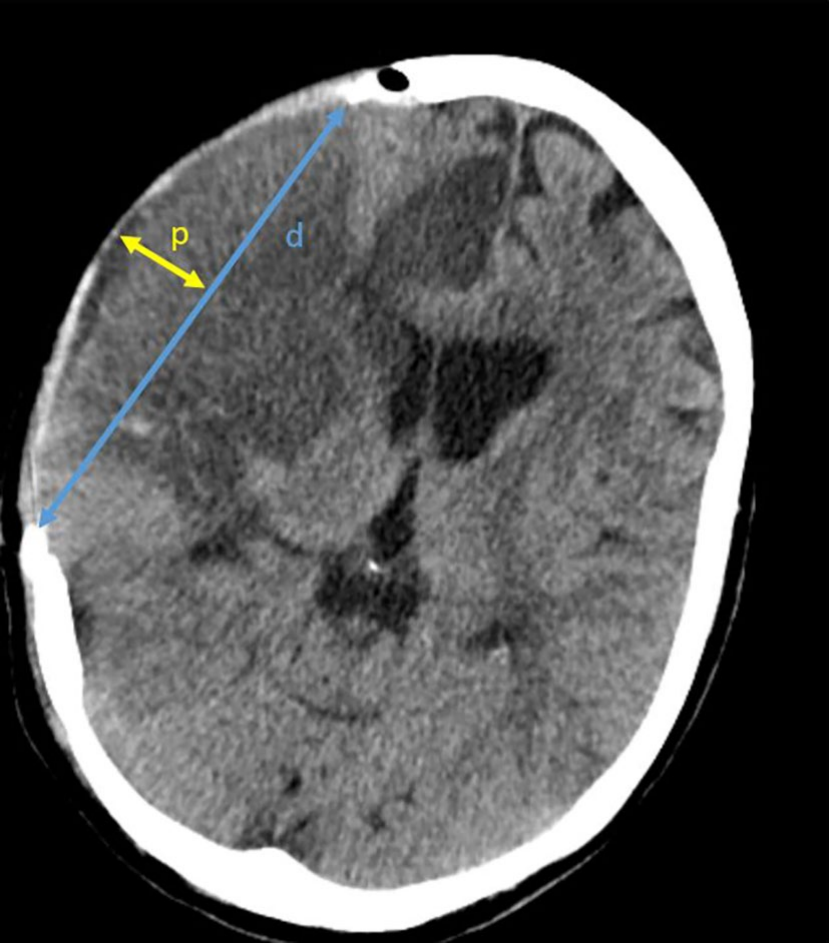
External brain herniation and decompression size assessed on postoperative CT scan. d was defined as the largest diameter of the craniectomy and p as the perpendicular line with the longest distance from the diameter to the dural flap at the same section.
